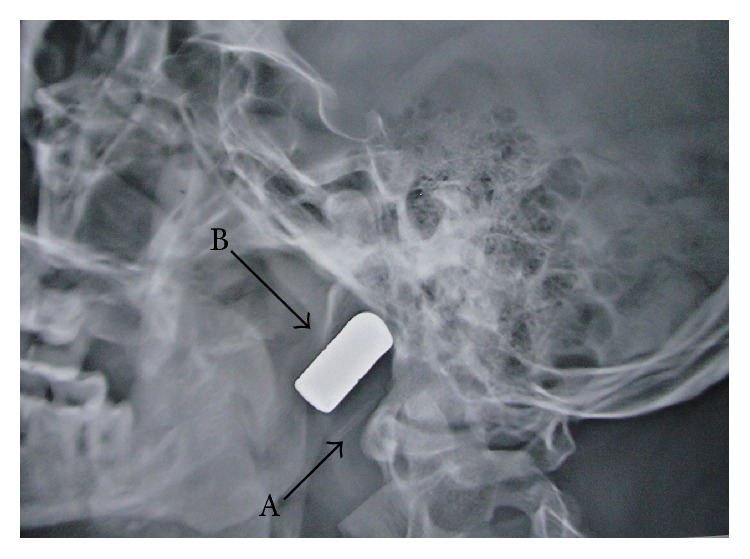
Preoperative X-ray of the skull, lateral view, showing the bullet (B) in the left parapharyngeal space at the level of the atlas (A).
